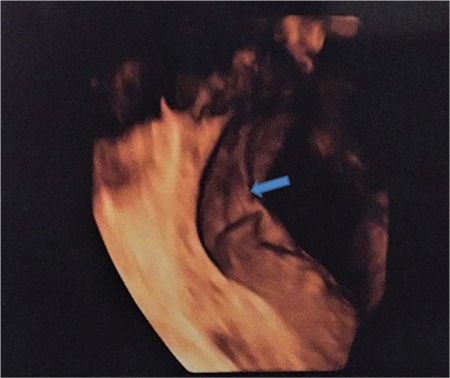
Intrauterine extra-amniotic adhesion demonstrated using 4D ultrasonography; “sheet on a string” appearance
